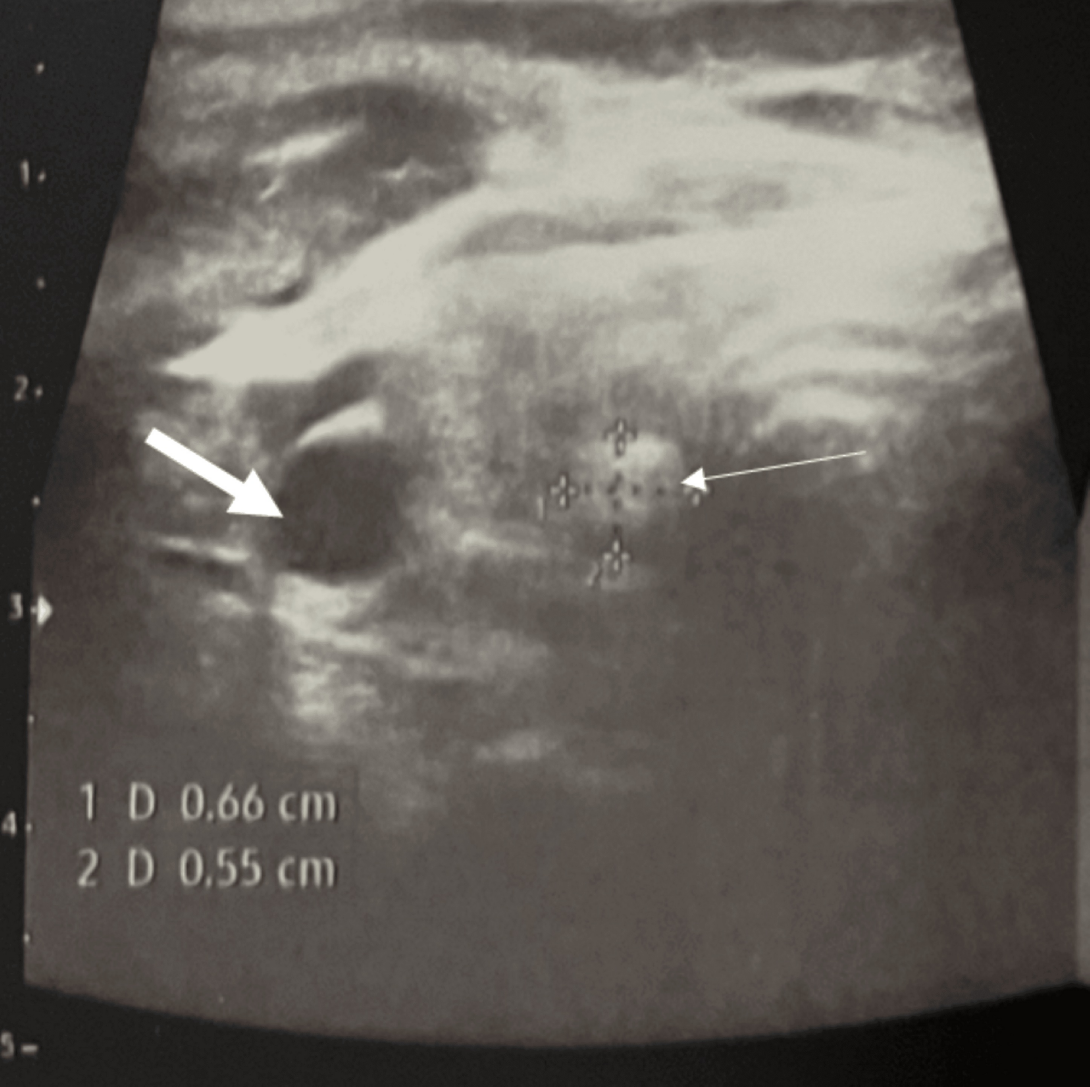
Thyroid ultrasound image showing an isthmic thyroid nodule (thin arrow) and a regenerative 'white knight' nodule (thick arrow) in the mid-portion of the right thyroid lobe
